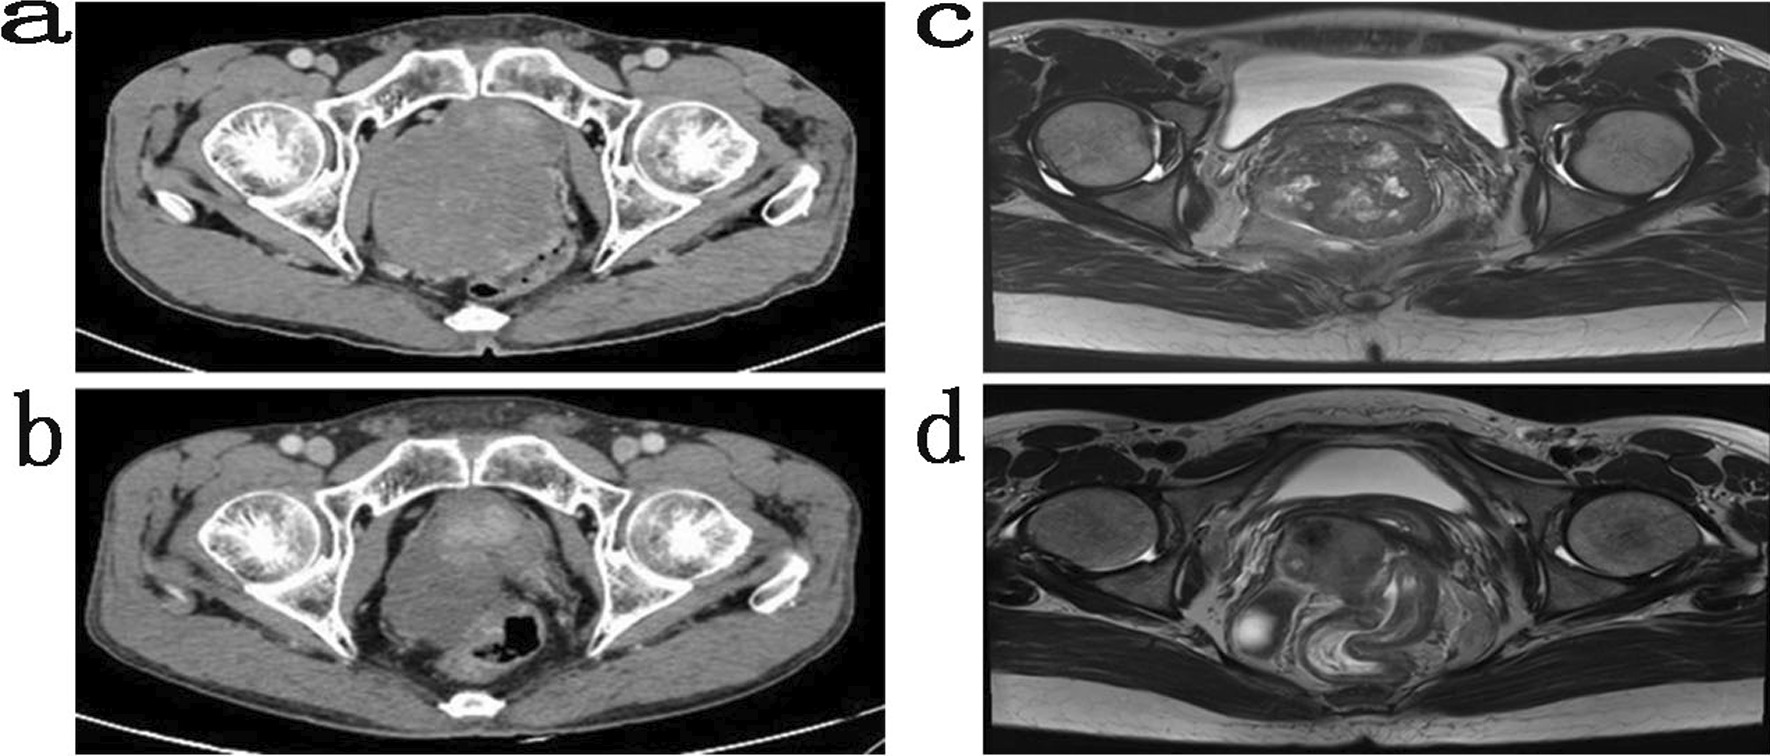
Imaging comparison of two patients before and after IM neoadjuvant treatment. Enhanced CT scan of the rectal GIST patient who received IM neoadjuvant therapy for 6 months (a initial tumor, and b tumor after 6 months of IM neoadjuvant therapy). Magnetic resonance imaging of another rectal GIST patient treated with IM neoadjuvant therapy for 9 months (c initial tumor, and d tumor after 9 months IM neoadjuvant therapy)
